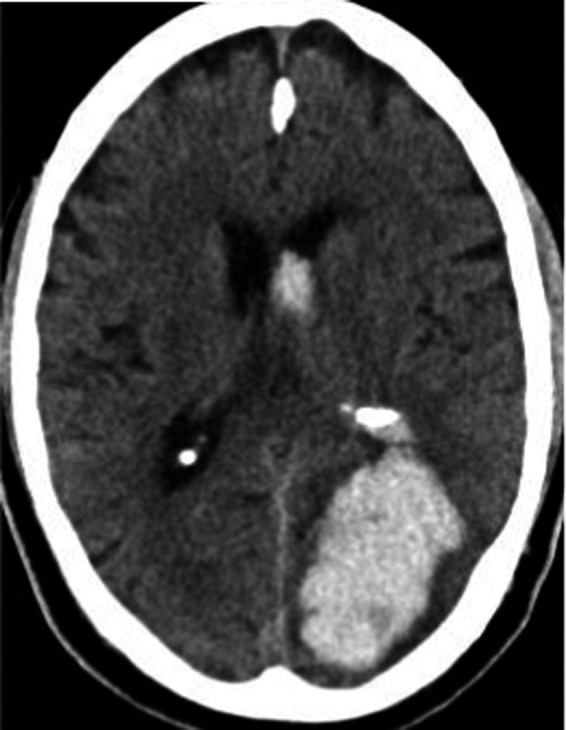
Atypically localized sICB with ventricular extension showed by brain CT scan. A 64‐year‐old woman who were presented with a fast‐progredient altered consciousness
